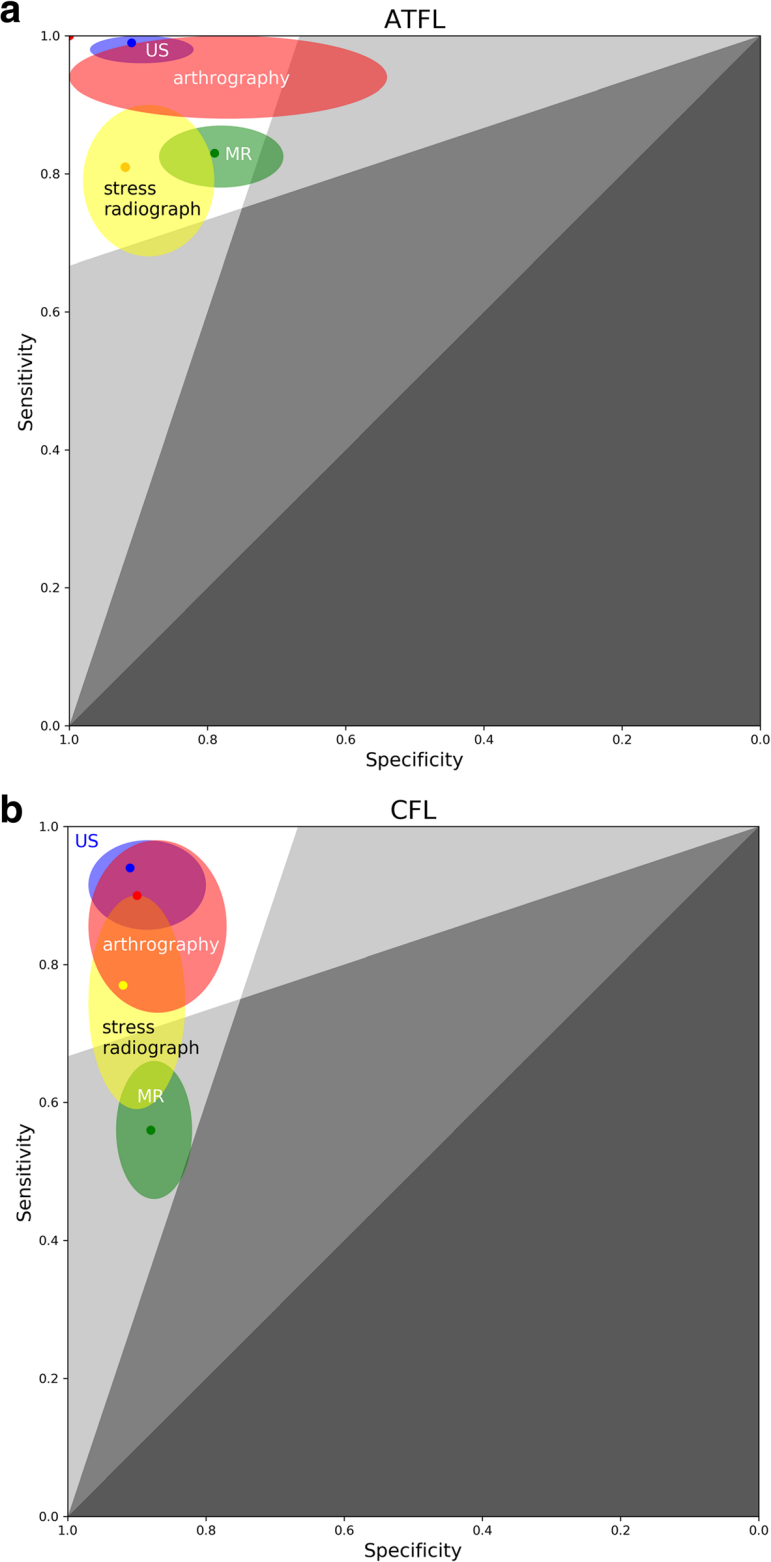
Zones of mostly bad imaging efficacy (ZOMBIE) plot for ATFL (a) and CFL (b). This is based on the receiver operating characteristic (ROC) plot. The colored dot stands for pooled sensitivity and specificity of each imaging technique, and the colored oval stands for 95% confidence interval of each imaging technique (green, MR; blue, US; red, arthrography; yellow, stress radiograph). Upper-left boomerang-shaped area formed by white and light grey zones defines zone of acceptable efficacy. Upper arm of the boomerang-shaped area stands for negative likelihood ratio below 0.33. Left arm of the boomerang-shaped area stands for positive likelihood ratio above 3
